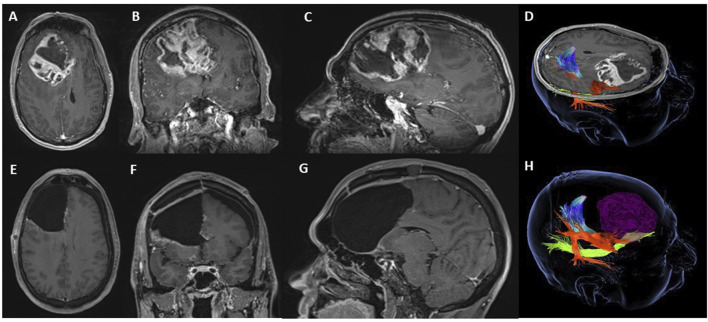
Case 2 right frontal contrast-enhancing lesion with a mixed cystic-necrotic component and invasion of the genu of the corpus callosum with minimal extension to the contralateral hemisphere and ipsilateral ependymal invasion causing a midline shift – preoperative T1-weighted images in the axial (A), coronal (B), and sagittal (C) planes. (D,H) show 3D models of the tumor and its relation to the subcortical white matter structures of interest: the corticospinal tract (multicolor), the arcuate fasciculus (orange), and the inferior fronto-occipital fasciculus (green). The Uncinate Fasciculus and Fronto-Aslant Tract were not reliably dissected using the constrained spherical deconvolution algorithm (Medtronic S8®). Postoperative images at 1 month of follow-up document complete resection of the contrast-enhancing component of this tumor with no complications - postoperative T1-weighted images in the axial (E), coronal (F), and sagittal (G) planes.
